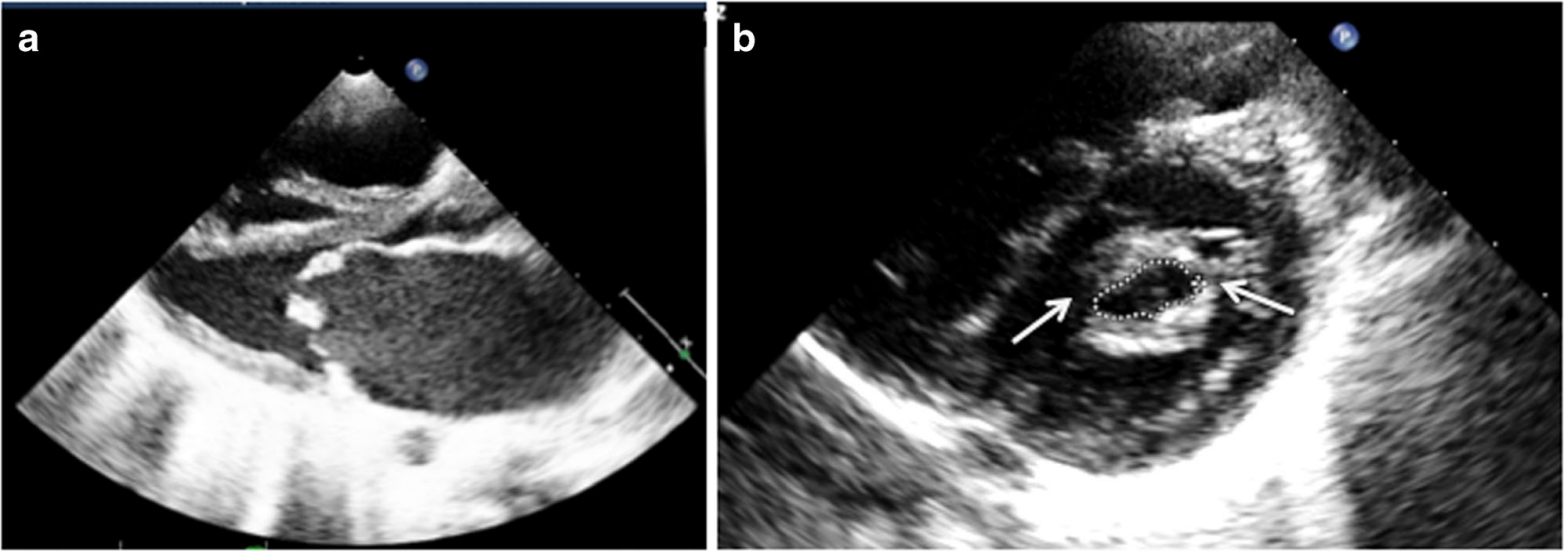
Echocardiogram from a patient with severe mitral stenosis showing thickened mitral valve with restricted opening (a) and commissural fusion (*arrows*) (b). Mitral valve area by planimetry is 0.73 cm^2^.
